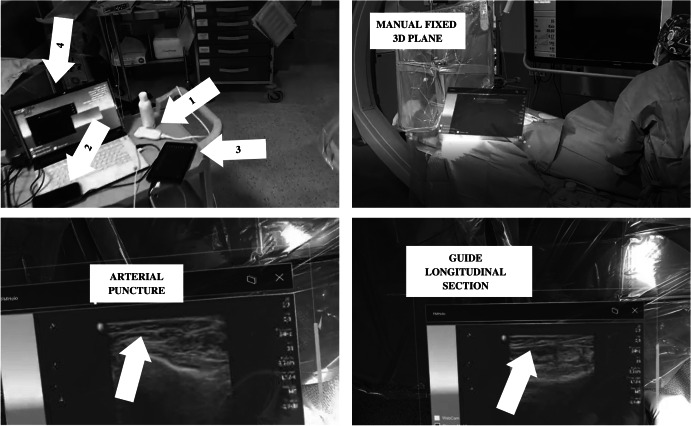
Different views of the system. Top left quadrant shows the main hardware components of the transmitter module: (1) ultrasound scanner, (2) video capture card, (3) tablet, and (4) laptop. Top right quadrant shows a plane rendering ultrasound scanner images fixed manually by the doctor over the patient using the button placed on the top right corner of the window (next to the cross button). Bottom-left quadrant shows an arterial puncture assisted by the system. Bottom-right quadrant shows the guide introduced into the artery from a longitudinal section view
